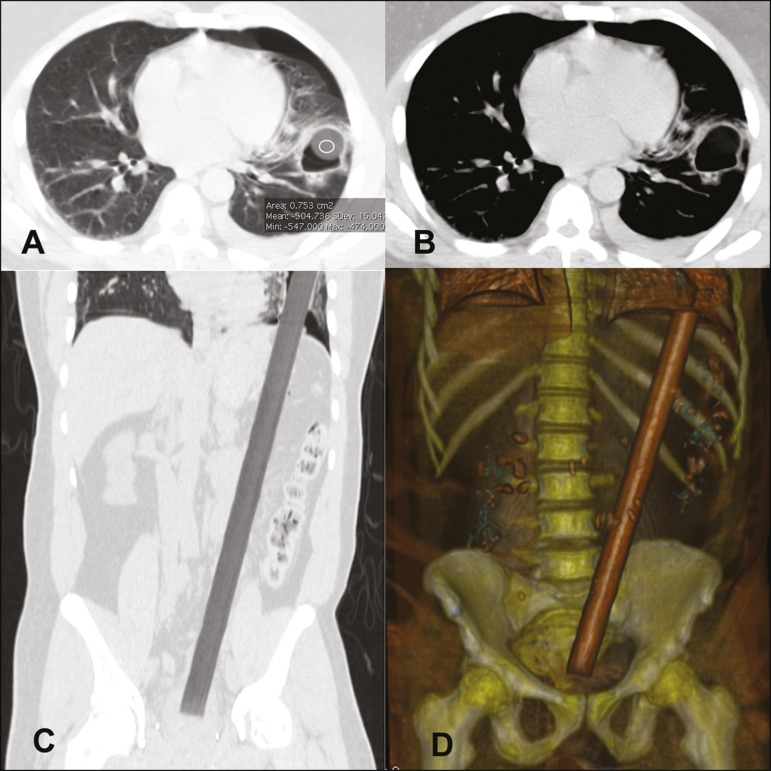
Axial CT with mediastinal and lung window settings (A and B, respectively)
